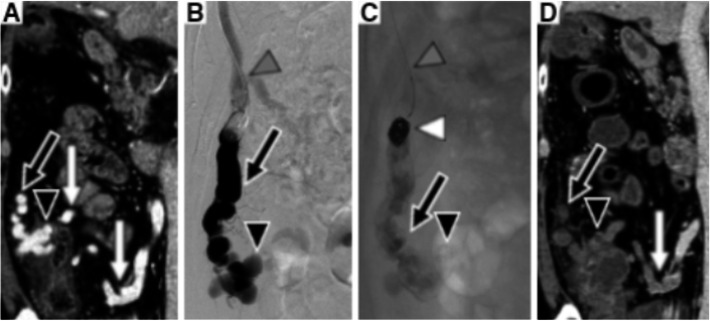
Close-up pictures of the ascending colonic varix. a Contrast medium-enhanced CT before B-RTO shows the ascending colonic varix (black arrowhead), the main draining vein (black arrow), and the ileocolic vein (white arrows) as a feeder vein. b B-RTV from the main draining vein (black arrow) shows the ascending colonic varix (black arrowhead) and microballoon catheter (gray arrowhead). c After injection of the sclerosing agent, microcoils (white arrowhead) are inserted via the microballoon catheter (gray arrowhead). When the balloon is deflated, contrast medium is retained in the colonic varix (black arrowhead) and the draining vein (black arrow). c corresponds to the enlarged image of Fig. 2b. d The day after B-RTO, contrast medium-enhanced CT shows no enhancement of the draining vein (black arrow), the colonic varix (black arrowhead), and the ileocolic vein (white arrow)
